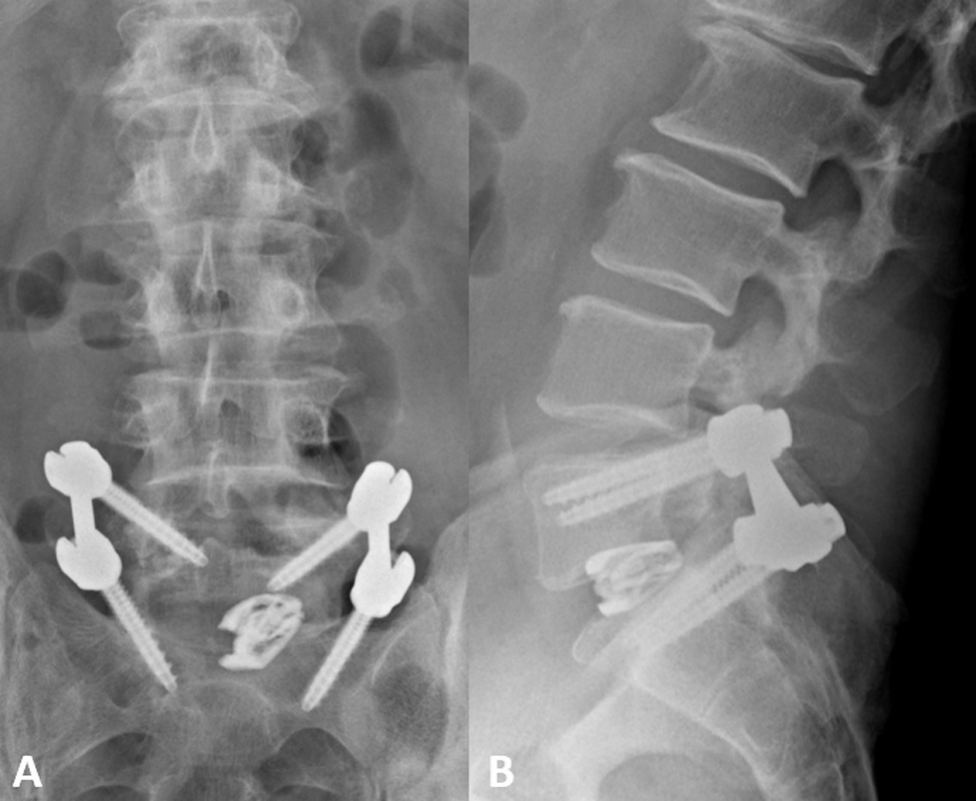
Postoperative lumbar spine X-ray 1 day after the procedure. (
